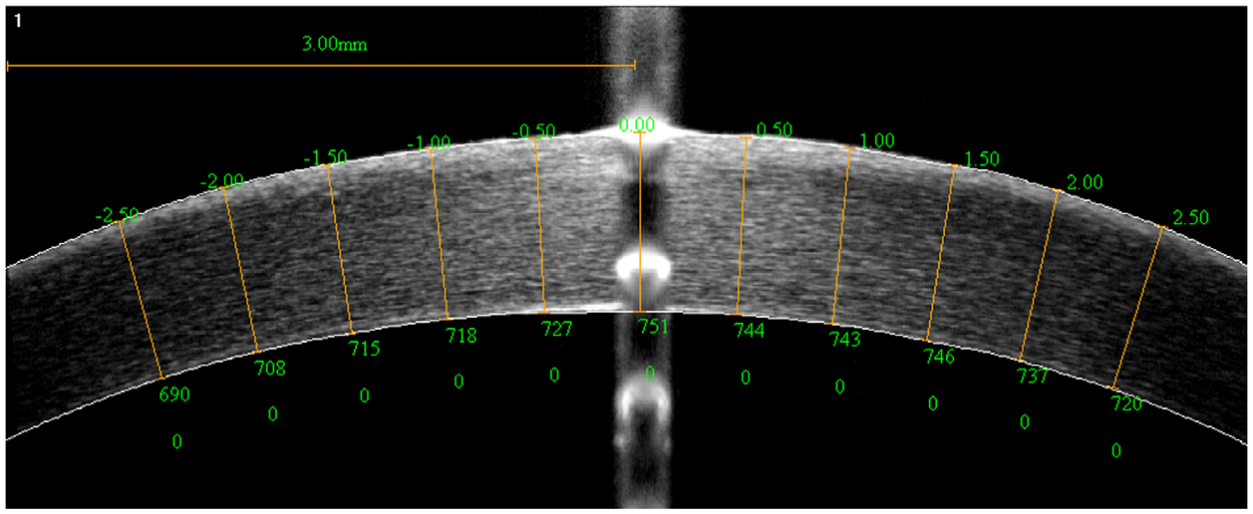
Pachymetry by OCT. Pachymetry was taken along the horizontal line of the suction ring using OCT. CT was measured on that meridian at 5 pairs of equidistant locations, ±0.5, ±1.0, ±1.5, ±2.0, and ±2.5 mm from the central spot, using the RTVue's “flap tool” in the CL-line scan.
